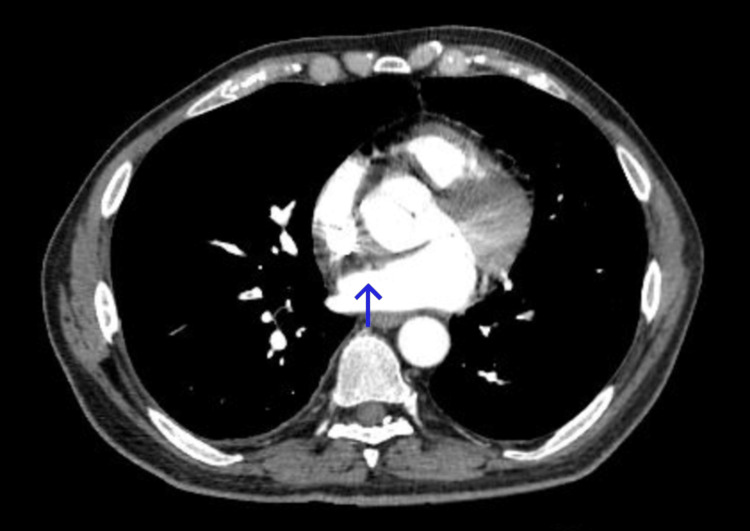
Computed tomography angiography of the chest showing a filling defect in the right lower lobe branch (arrow)
